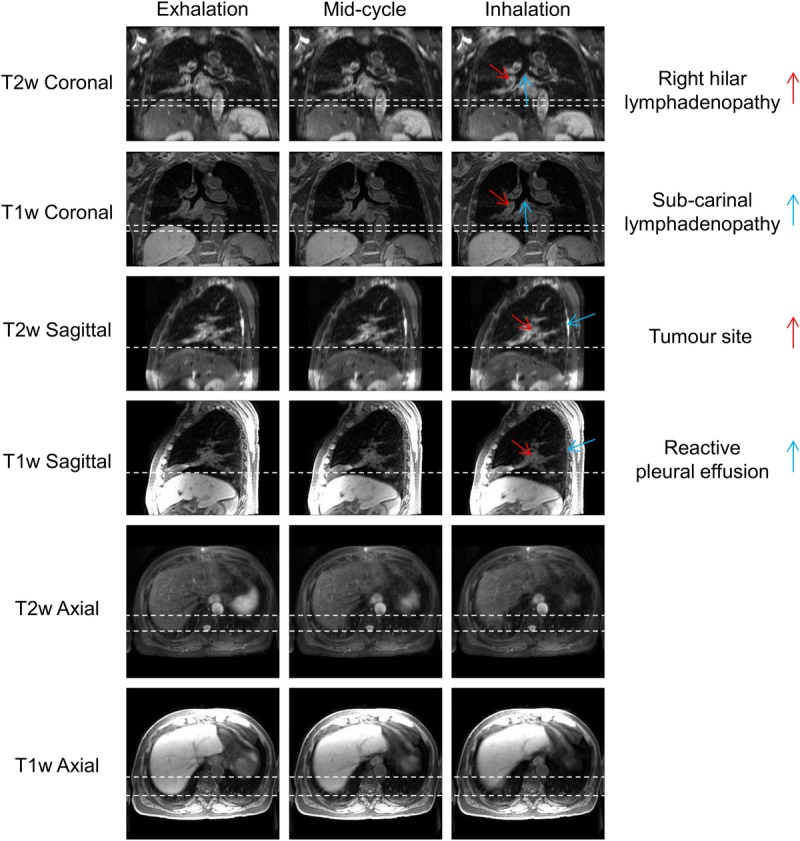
Example T1w and T2w coronal, sagittal, and axial views at exhalation, midcycle, and inhalation respiratory phases for patient 7. This patient was diagnosed with T1aN2 primary lung squamous cell carcinoma and had a small benign reactive pleural effusion. The dashed white lines aid visualization of the diaphragm surface position. The respiratory pattern of T1w MRI is preserved in T2w MRI. The tumor site, lymphadenopathy, and reactive pleural effusion are shown more clearly in T2w MRI than T1w MRI.
